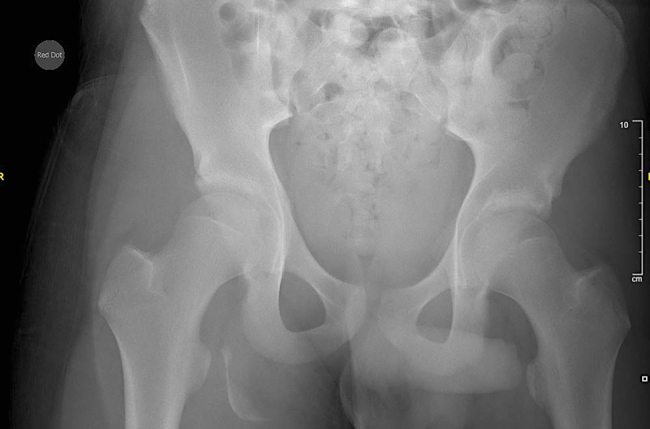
Preoperative anteroposterior pelvic radiograph showing the displaced right ITAF. ITAF = Ischial tuberosity avulsion fracture.
